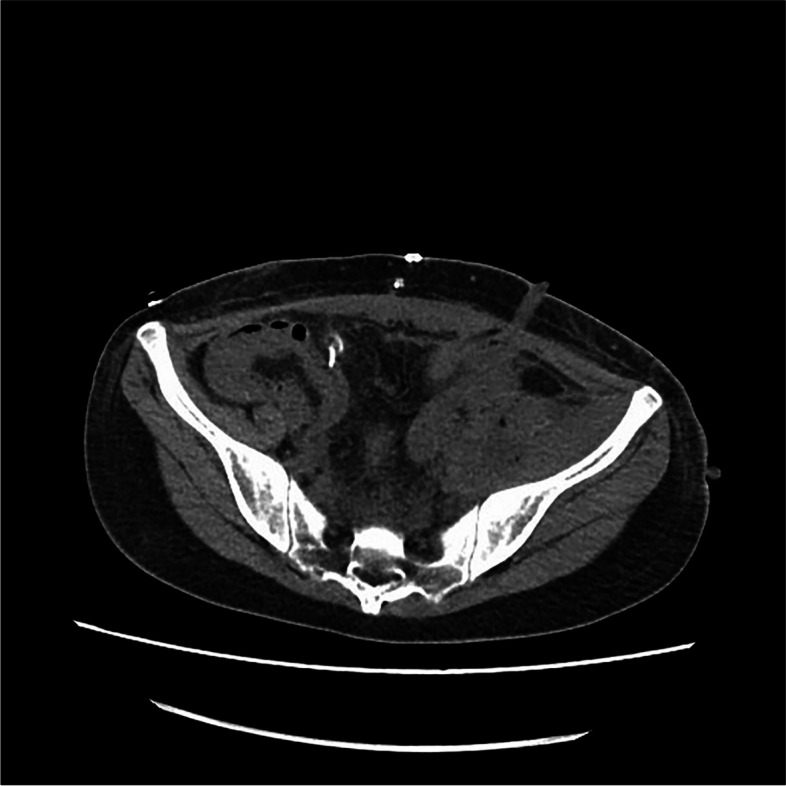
The CT scan taken shortly after her tumor rupture in her retroperitoneum with drainage tube after Emergent debridement surgery (patient number 5 in Table 2). Following debridement and suturing, this female patient recovered from the infection and continued using single chemotherapy of irinotecan
